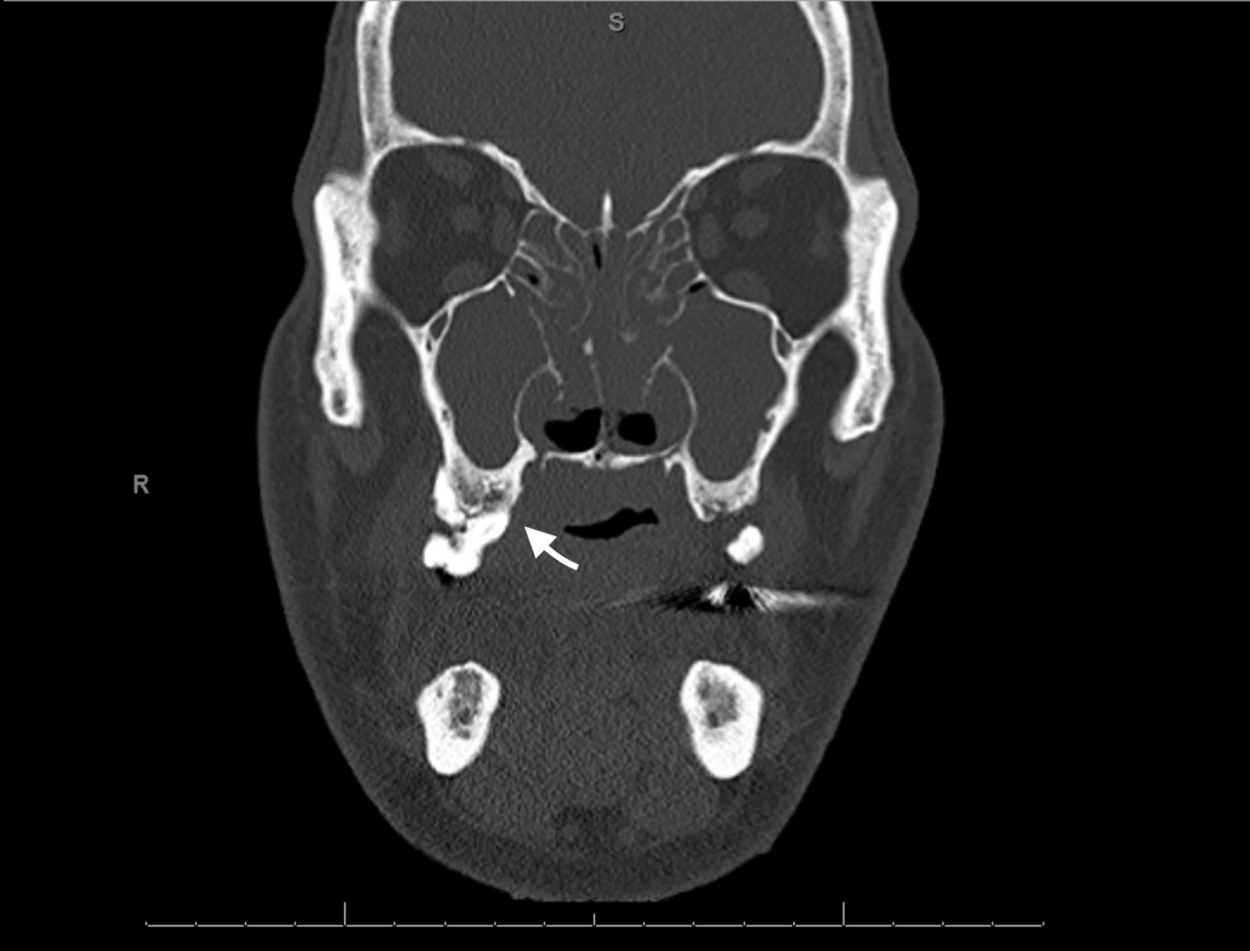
CT maxillofacial w/o contrast on presentation
